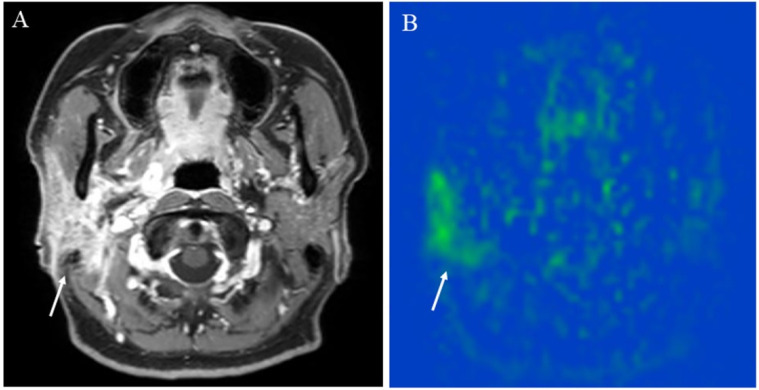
A 48-year-old female with right chronic parotitis: (A) Contrast-enhanced T1-weighted image shows intense right parotid gland enhancement (arrow); (B) pCASL image shows higher blood flow within the lesion (arrow) (right mean of 81.17 mL/100 g/min) than the opposite parotid gland (left mean of 36.82 mL/100 g/min).
